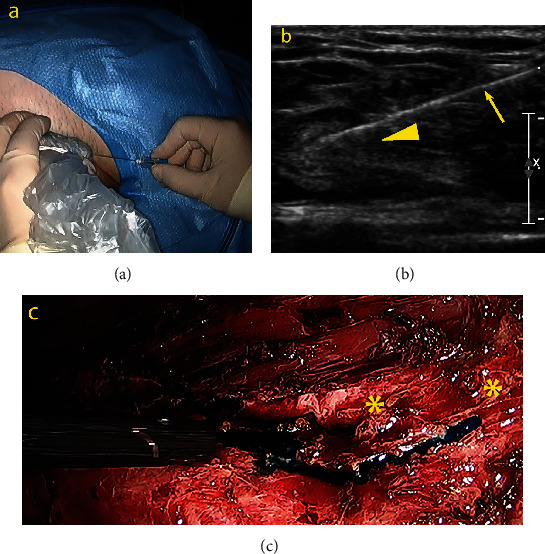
Identification of the point of chylous leakage. External (a) and ultrasound vision (b) of a transnodal injection of methylene blue under image guidance (lymph node, head arrow; needle, and arrow). Two leak points (stars) can be observed in the retroperitoneum (c) after seven minutes of percutaneous administration of methylene blue.
